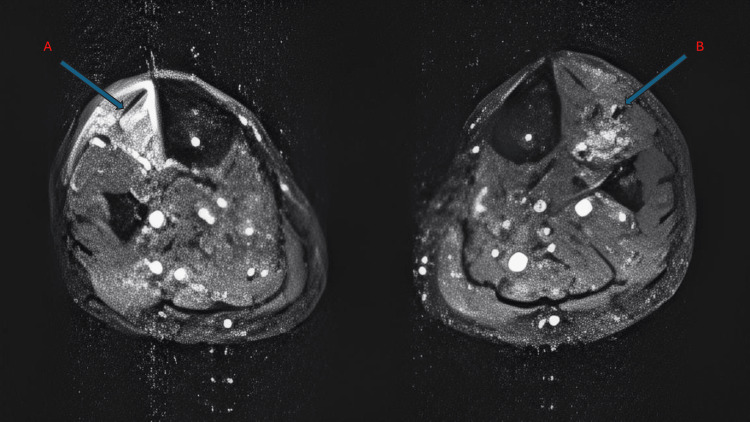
MRI of the lower limbs (A) Cross-section through the left leg showing the tibialis anterior (arrow), with increased signal intensity consistent with oedema and evidence of reduced muscle bulk suggesting early atrophy.
